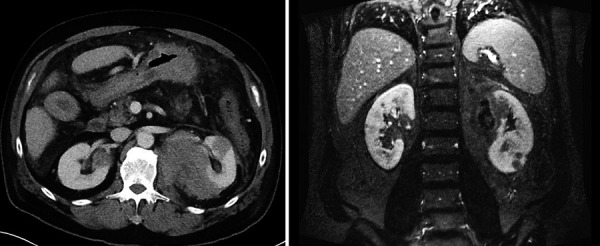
Abdominal MRI. Multiple rounded hypo enhancing lesions noted throughout the renal parenchyma bilaterally. Axial view (left). Coronal view (right).
